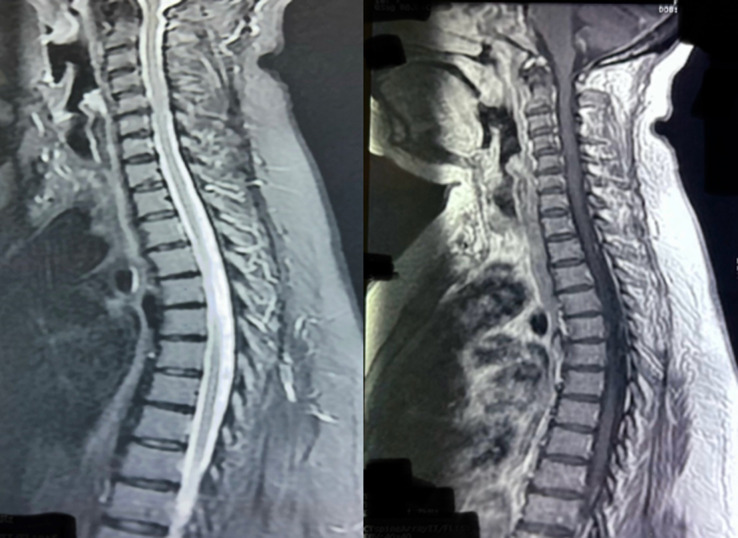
magnetic resonance imaging showing syringomyelic cavity
